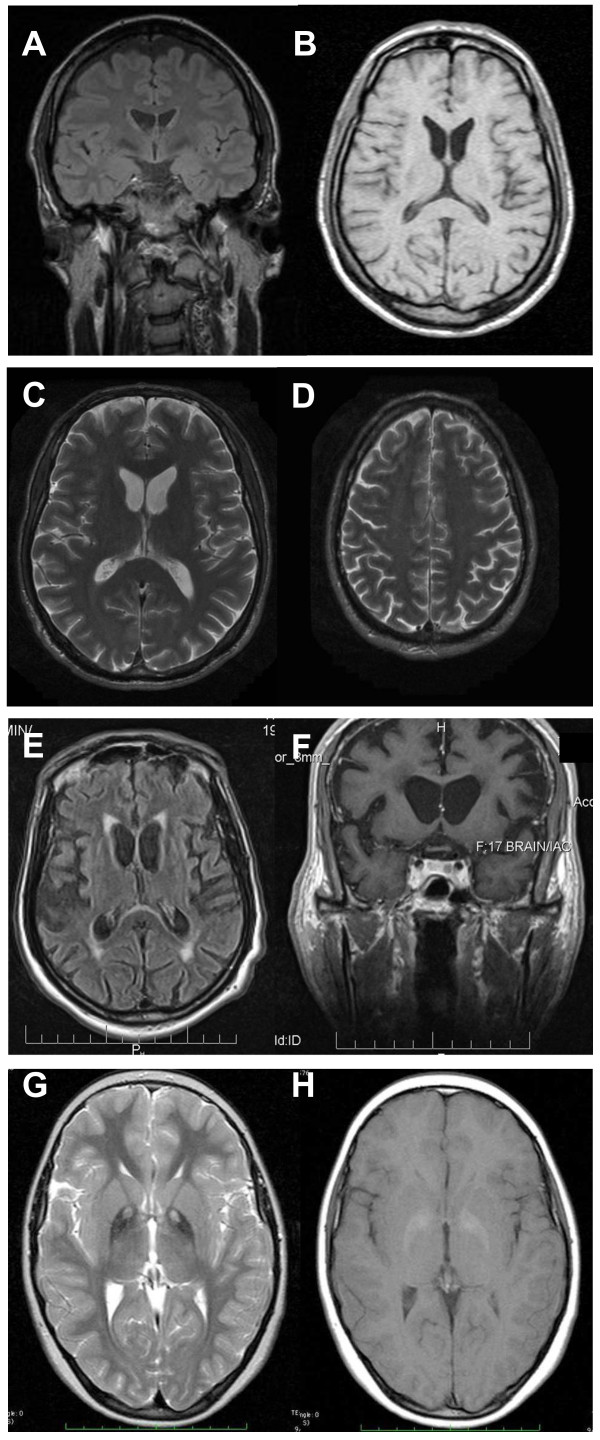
Neuroimaging. *ChAc. *Coronal FLAIR- (A) and axial T1-weighted (B) images demonstrate moderate atrophy of the caudate nucleus. *MLS. *Axial T2-weighted images demonstrate moderate atrophy of caudate nucleus and putamen (C) but no relevant cortical atrophy (D). *HDL2. *Axial FLAIR- (E) and coronal T1-weighted images (F) demonstrate atrophy of the caudate nucleus and the fronto-temporal cortex. In addition, FLAIR images show periventricular white matter hyperintensities (courtesy of Nora Chan, MD, UCLA, Los Angeles, USA). *PKAN. *T2-weighted fast spin echo (G) and T1-weighted (H) brain MRI scans from a child with PKAN demonstrating the "eye of the tiger" sign (courtesy of Susan J. Hayflick, MD, Oregon Health and Science University, Portland, Oregon, USA)
